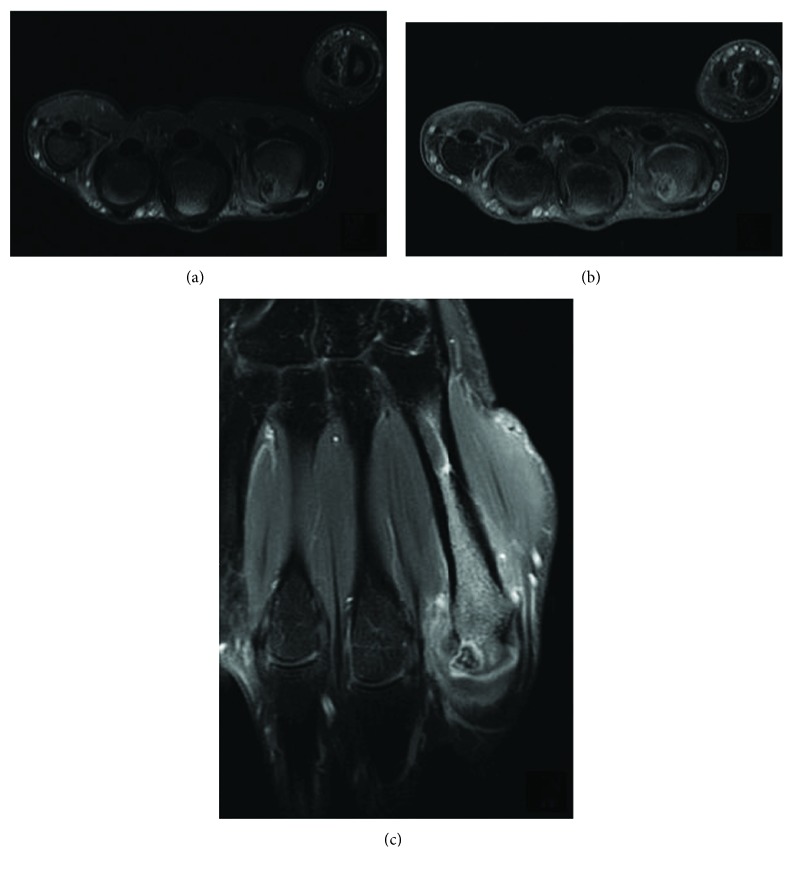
Nuclear magnetic resonance imaging (NMRI). (a) Axial fat-suppressed T2-weighted NMRI. (b, c) Respectively, axial and coronal, gadolinium-enhanced T1-weighted NMRI.
